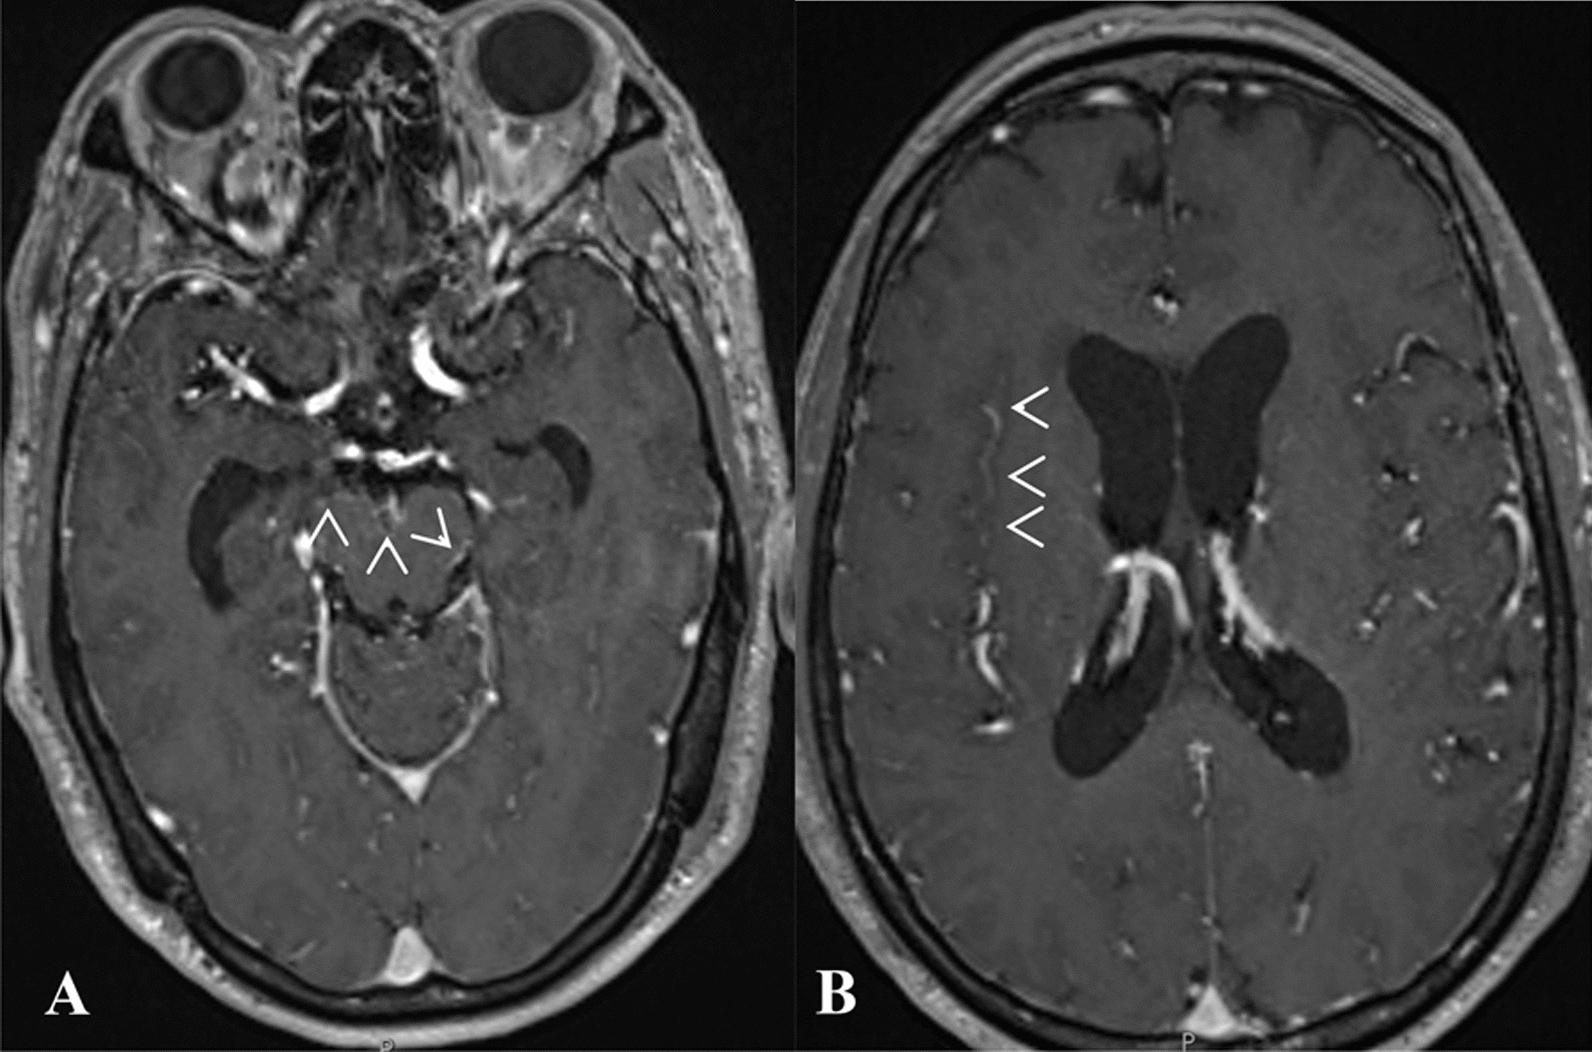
Hydrocephalus and leptomeningeal enhancement on magnetic resonance image on day 6 (re-analysis). Contrast enhanced axial T1-weighted images (A, B) reveal hydrocephalus with dilated frontal and temporal horns of lateral ventricles. Leptomeningeal enhancement noted (arrowheads) at basal cistern, ambient cistern (A) and the right Sylvian fissure (B)
